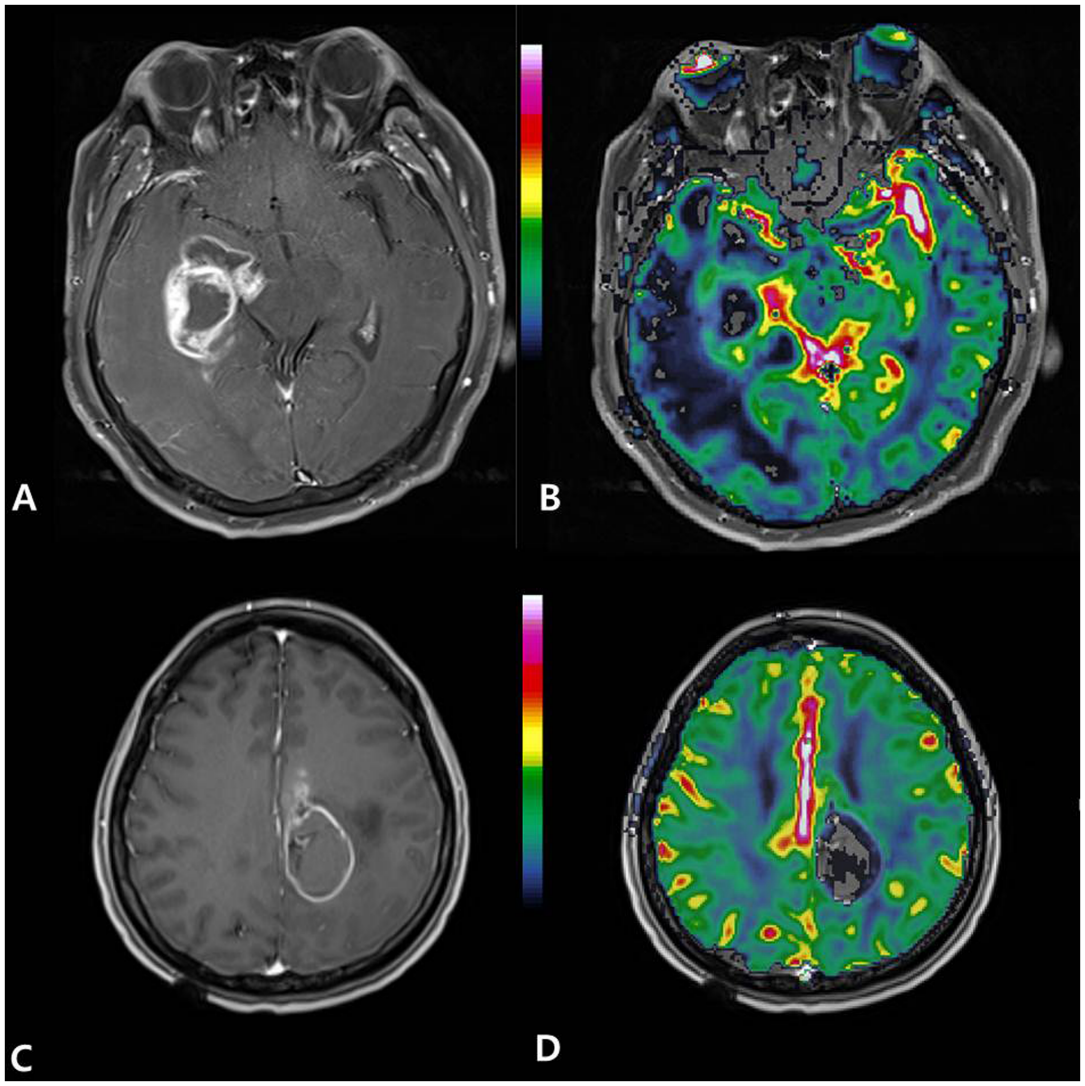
Glioblastomas with favorable genetic profiles show low normalized relative tumor blood volume (nTBV). (A, B) A 67-year-old man had glioblastoma with EGFR expression (2+), PTEN loss (−), MGMT methylation (+), and a Ki-67 index of 5%. The tumor showed low nTBV (4.3). (C, D) A 36-year-old woman had glioblastoma with EGFR expression (+), PTEN loss (−), MGMT methylation (+), and a Ki-67 index of 9%. The tumor showed low nTBV (4.59) (A, C) Contrast-enhanced T1-weighted axial image, (B, D) normalized relative cerebral blood volume (nCBV) map overlaid on structural contrast-enhanced T1-weighted axial image.
